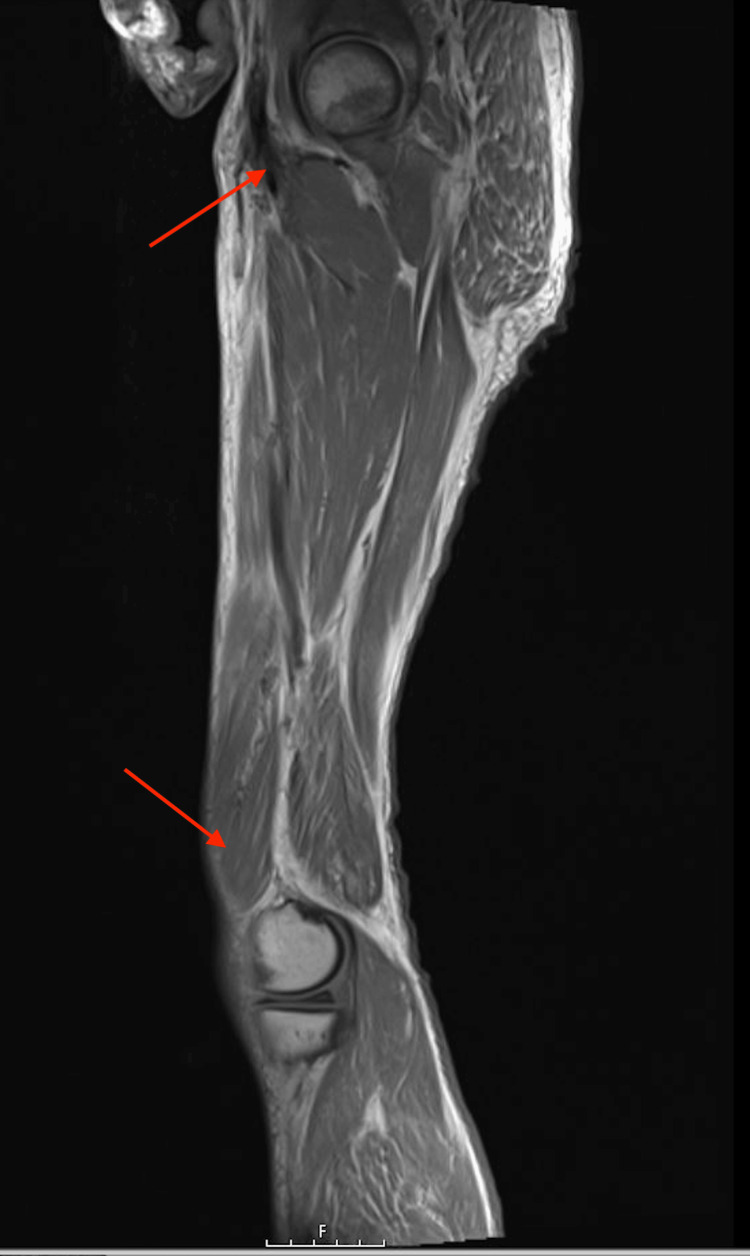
MRI right lower extremity non-joint without contrast should diffuse a decreased T1 and increased T2 signal within the subcutaneous adipose tissue (red arrow). MRI: magnetic resonance imaging.
